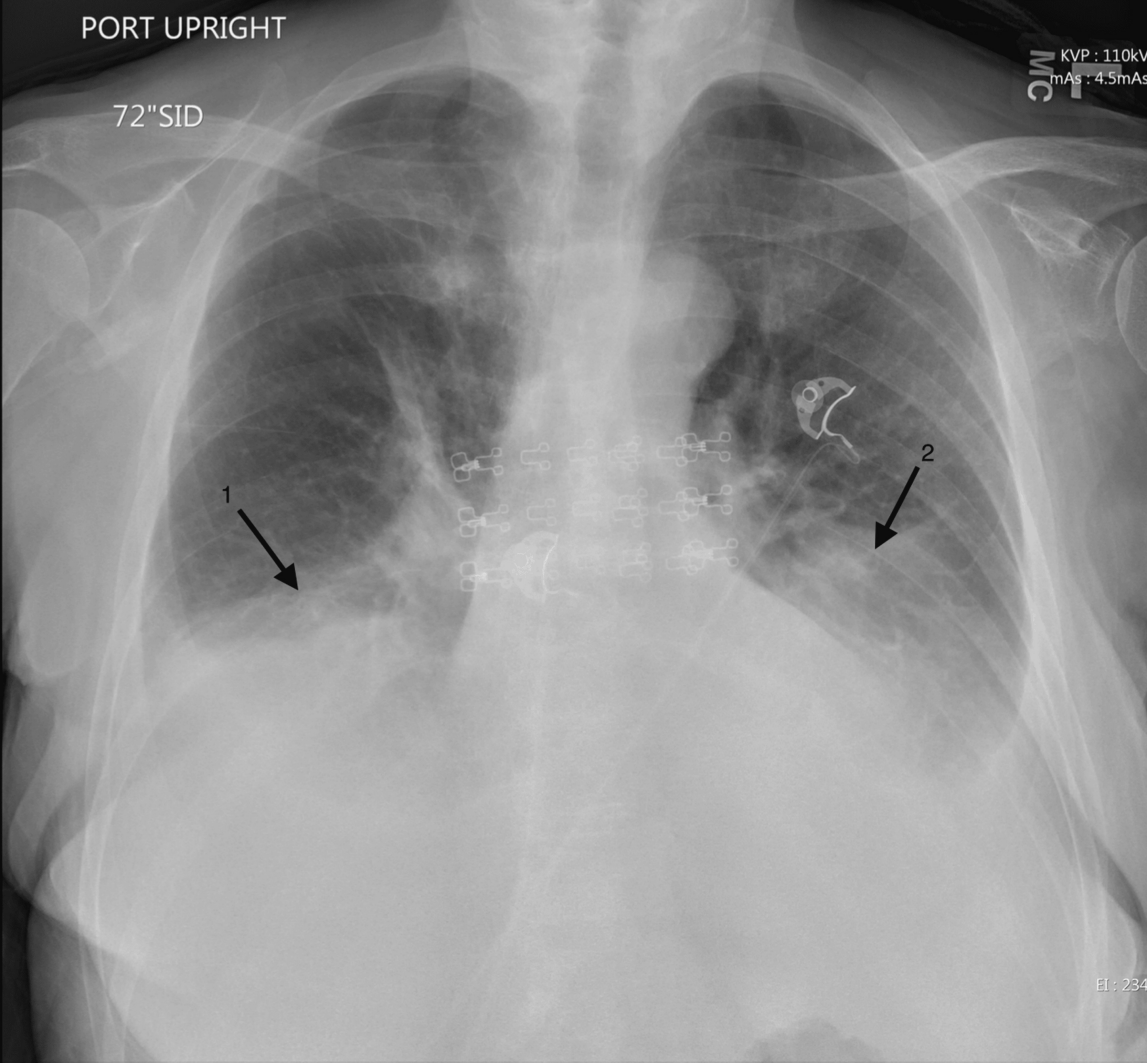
Chest radiograph on initial presentation identifying bilateral pleural effusions Arrows 1 and 2 display left and right pleural effusions, respectively. No pneumothorax was visualized
